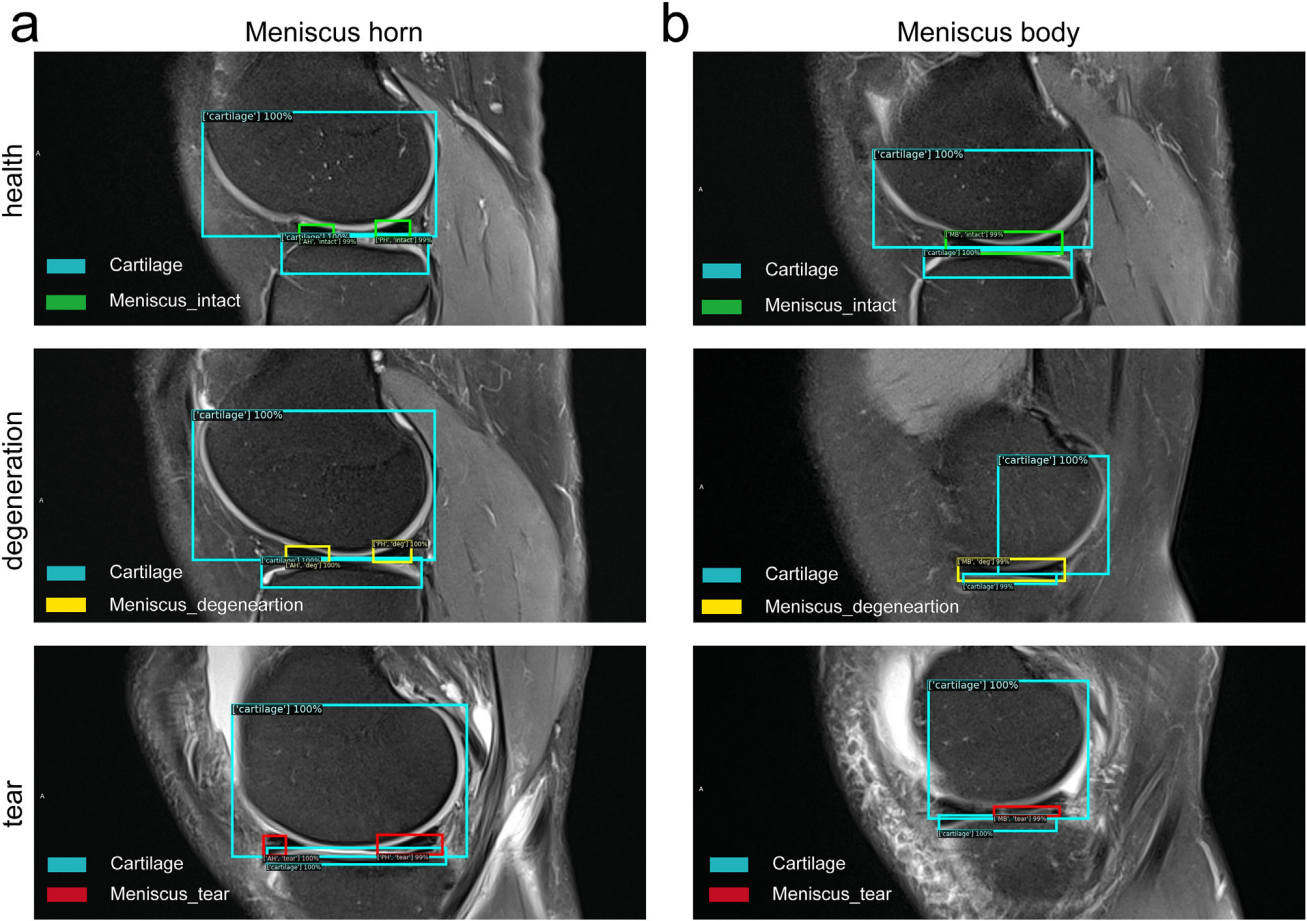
Bounding box diagnosis results on meniscus MR images. (a) Meniscal horns, (b) Meniscal body.
